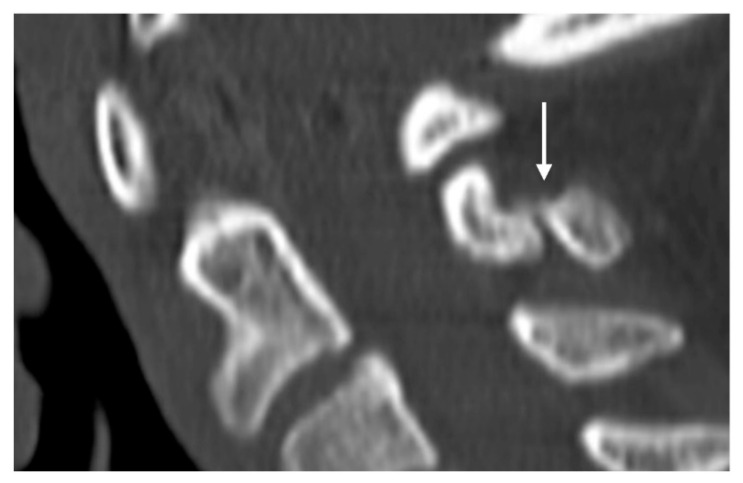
Sagittal CT image demonstrating a non-united fracture of C2 spinous process (white arrow).
